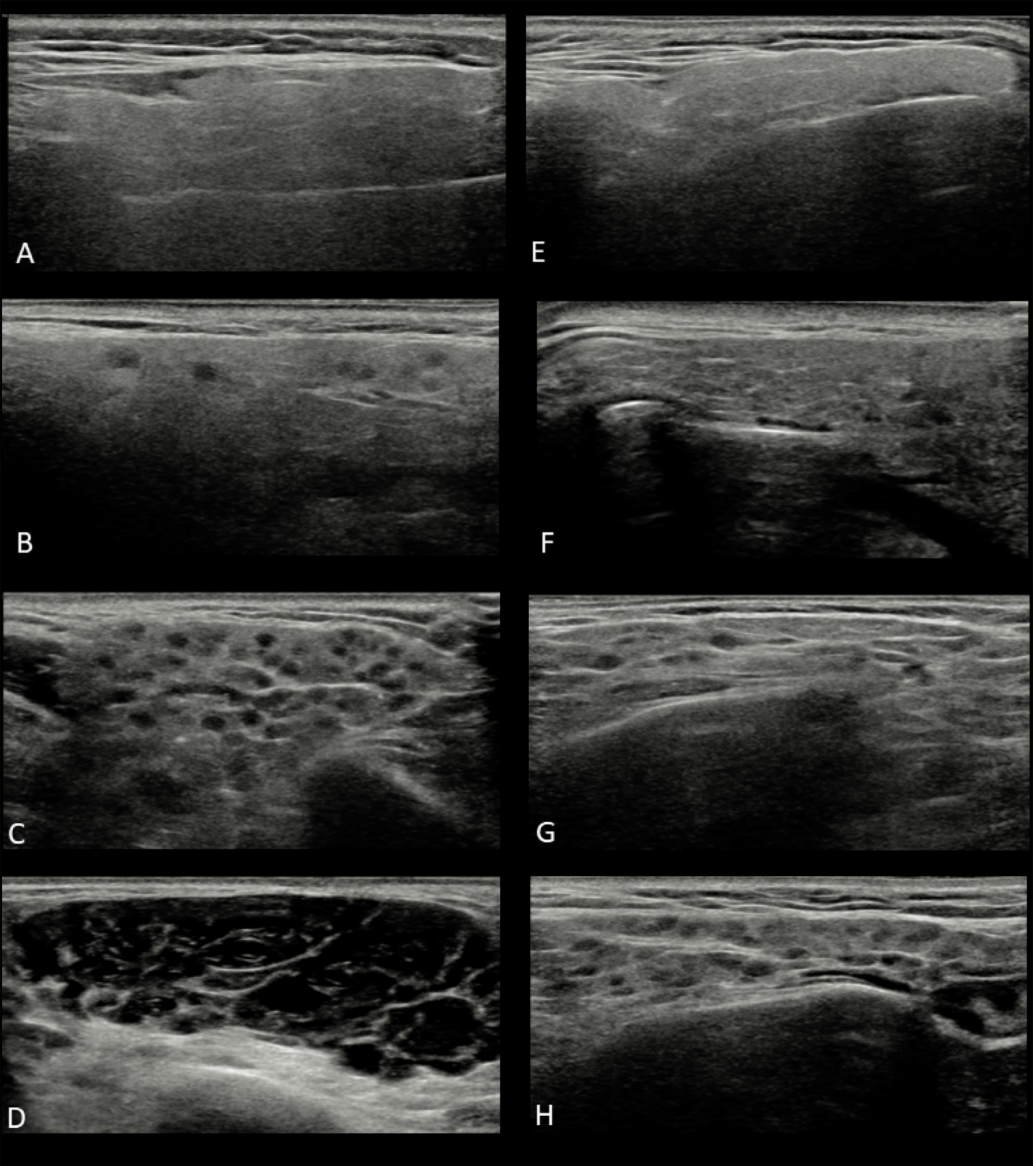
Ultrasound images of parotid glands in the two four-grade semi-quantitative scoring system: (A) De Vita et al. score grade 0; (B) De Vita et al. score grade 1; (C) De Vita et al. score grade 2; (D) De Vita et al. score grade 3; (E) OMERACT score grade 0; (F) OMERACT score grade 1; (G) OMERACT score grade 2; (H) OMERACT score grade 3.
